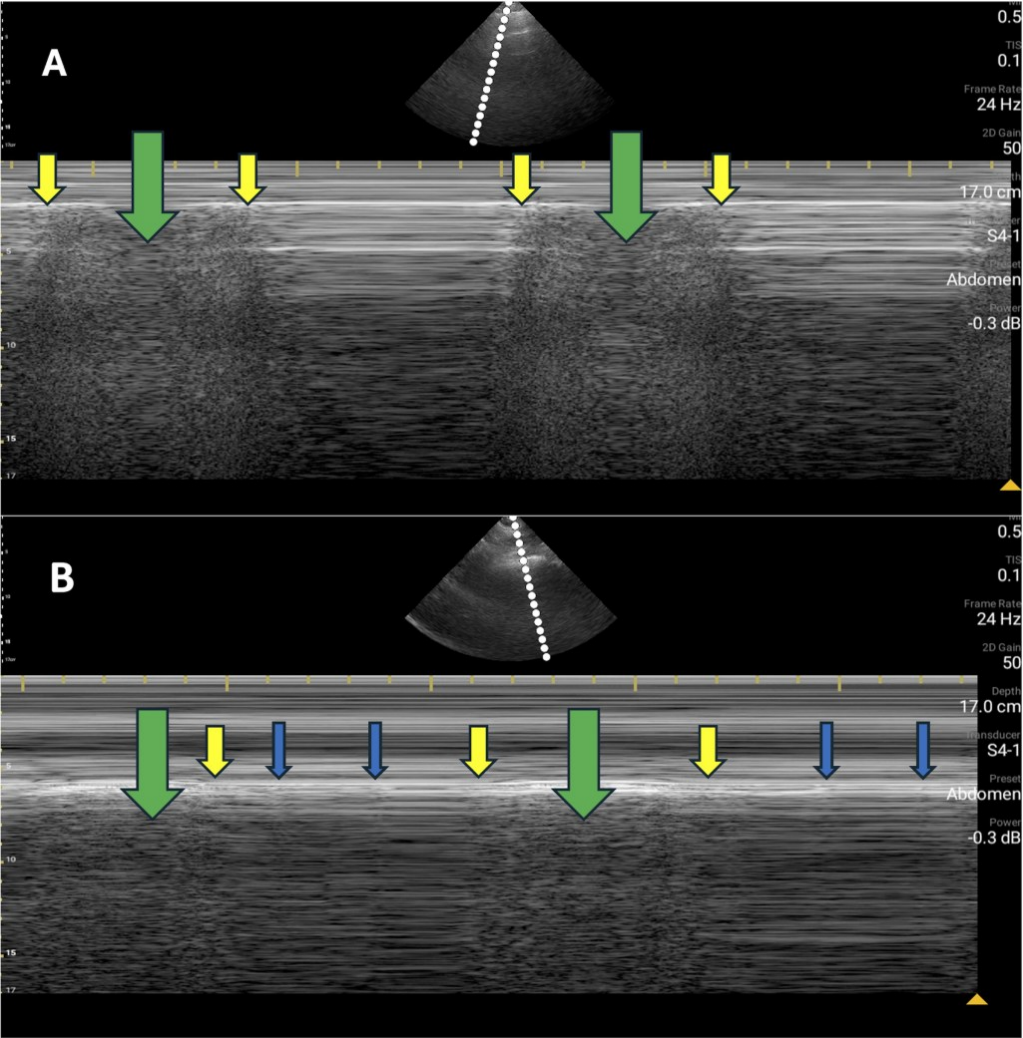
Point of care ultrasound (POCUS) exam in M-mode demonstrating alternating “bar-code sign” and “sandy-shore sign.” Panel A depicts imaging at the left lung apex (at the midclavicular line in the second intercostal space). Panel B depicts imaging at the right axilla (at the right anterior axillary line in the fourth intercostal space). The yellow vertical arrows point to the transition between present and absent lung sliding. The tip of the yellow arrow rests on the pleural line. The green vertical arrows point to normal lung sliding. The blue arrows (Panel B) indicate lung pulse present in the bar-code regions.
